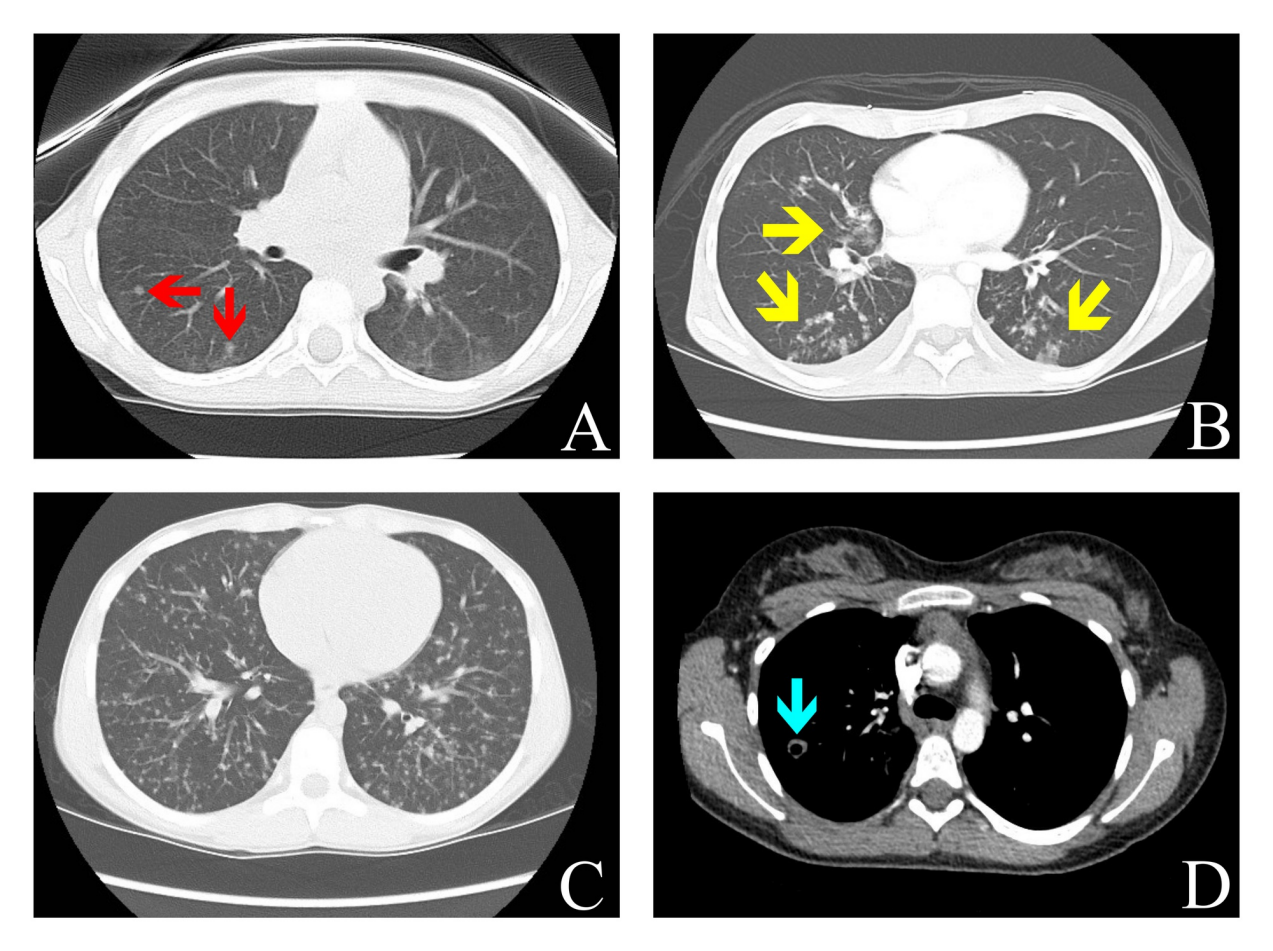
Representative computed tomography (CT) images of patients with pulmonary manifestations of inflammatory bowel disease. A: Case 2 with two representative nodules in the right lung (red arrows); B: Case 3 with extensive, bilateral tree-in-bud opacities (yellow arrows); C: Case 4 with diffuse pulmonary micronodules; D: Case 5 with nodule with central cavitation in the right lung (blue arrow).
